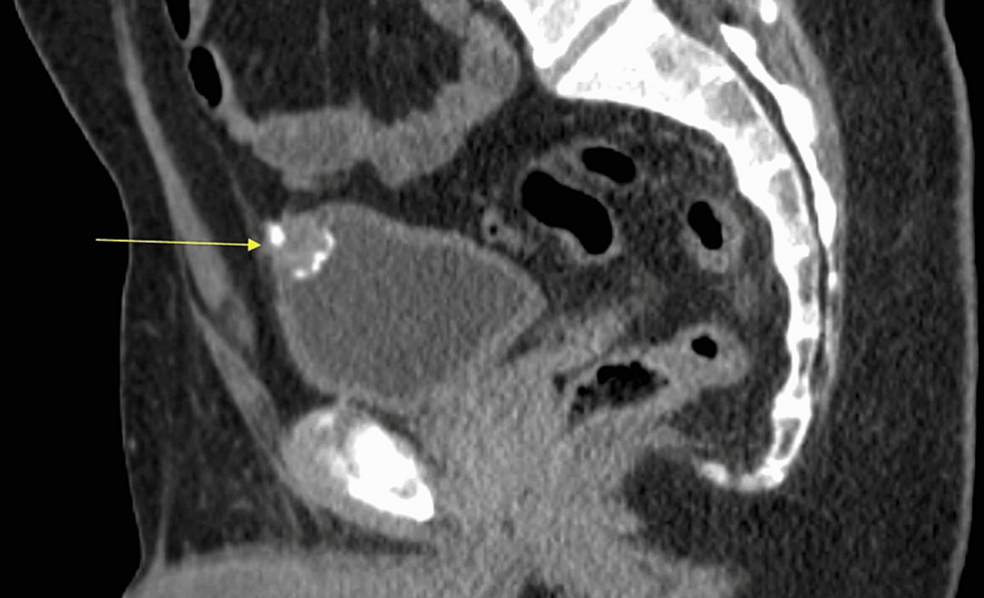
CT Urogram with automatic exposure control (AEC) indicating 1.9cm upper anterior bladder wall mass suspicious for a carcinoma.
